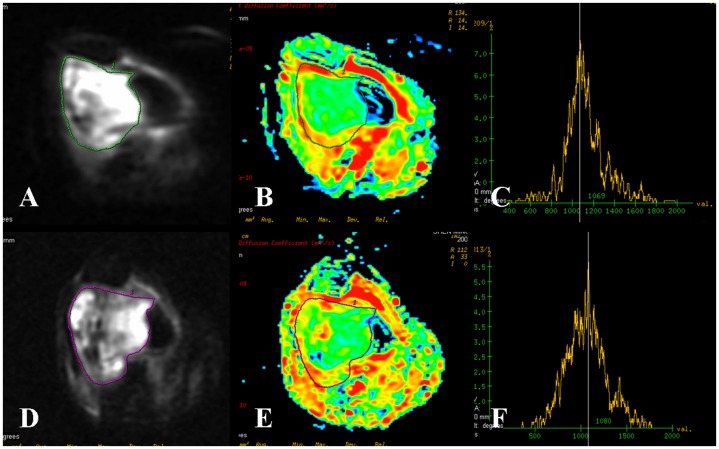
Osteosarcoma of the distal femur in a 46-year-old woman with poor response. (A∼C) DWI map, ADC map and ADC histogram before neoadjuvant chemotherapy. The tumor on DWI map was mixed high signal intensity. The ADC value of the whole tumor was 1.35×10^−3^ mm^2^/s with a subtotal green area. The ADC histogram was high and sharp. (D∼F) DWI map, ADC map and ADC histogram after neoadjuvant chemotherapy. The tumor on DWI map was mixed signal intensity. The ADC value of the whole tumor (1.31×10^−3^ mm^2^/s) and the ADC histogram were similar to those of pre- neoadjuvant chemotherapy. Tumor necrosis rate of 20% was confirmed by postoperative pathological evaluation. The effectiveness of neoadjuvant chemotherapy was poor.
